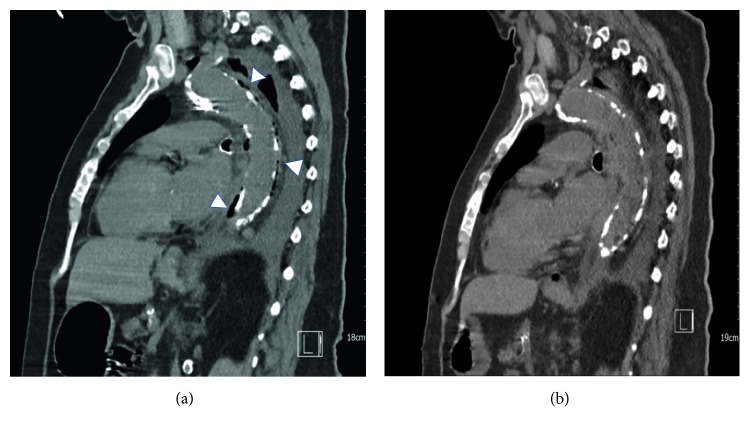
Sagittal view on chest CT obtained on admission (a). Note the presence of periaortic gas and inflammatory thickening of the aortic wall (arrowheads) without aneurysmal dilation, extending along the aortic arch and descending aorta. By admission day 10, CT findings showed resolution of the periaortic emphysema with interval development of nonspecific fluid and inflammatory changes (b).
